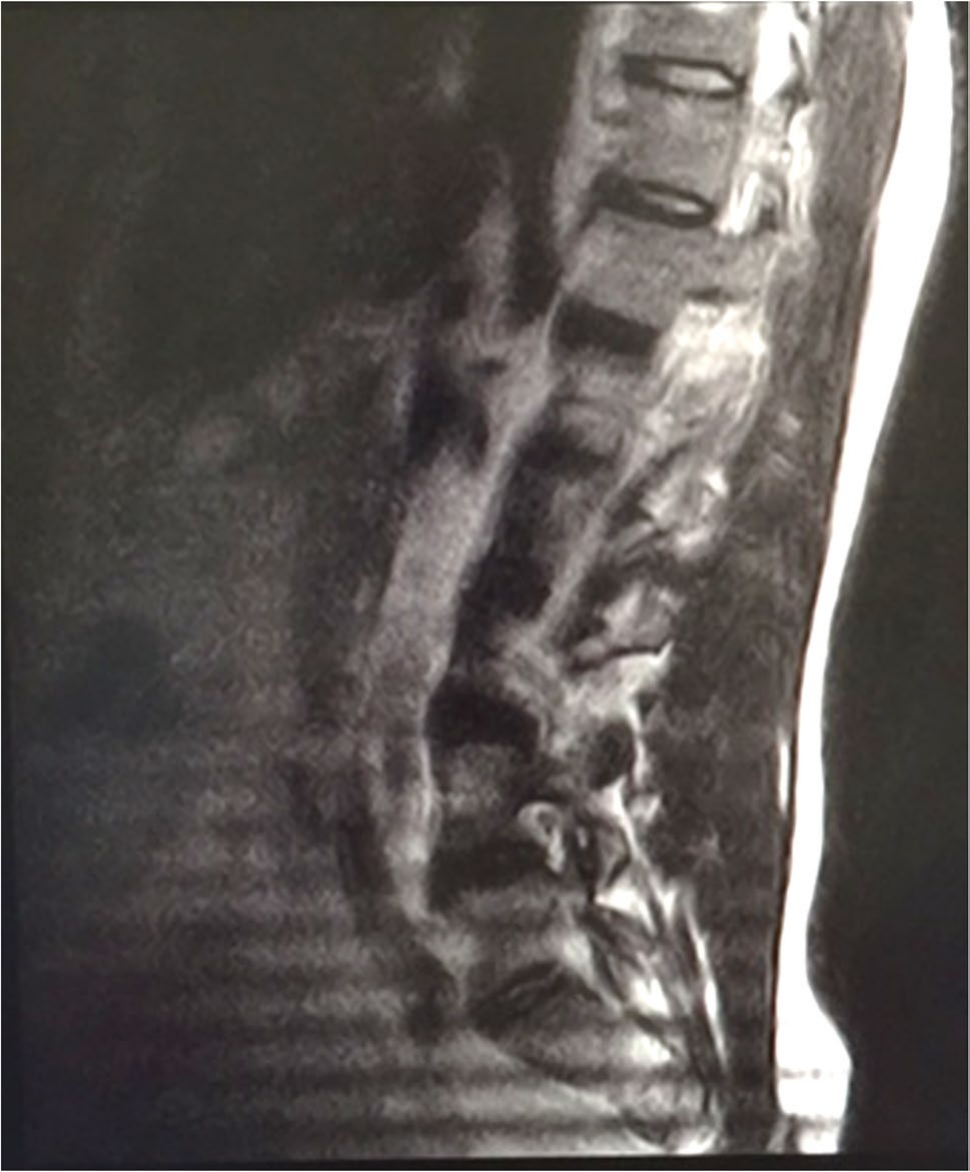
Example of decreased image quality due to episodic technical issues with the 0.5 T upright MRI unit
